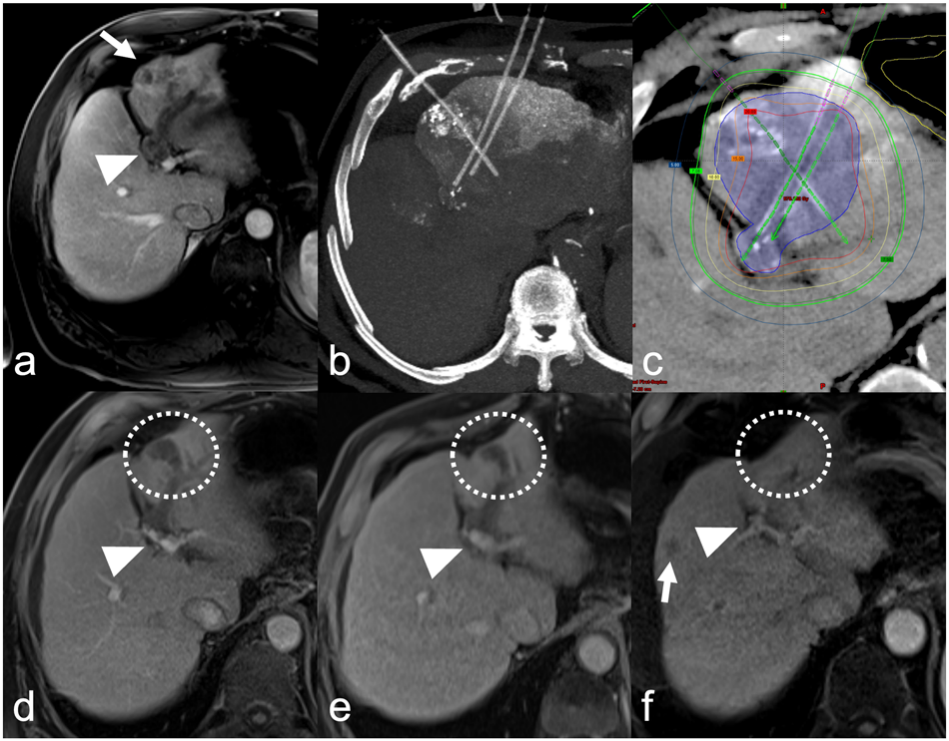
Example images in a 71-year-old male treatment-naive patient with unresectable hepatocellular carcinoma (HCC). a Baseline MRI scan (portal venous phase) shows a single 63-mm HCC (arrow) with portal venous tumor thrombus in the left first-order branch (VP3, arrowhead) in the left liver lobe. b Maximum intensity projection of a computed tomography scan acquired after placement of three brachytherapy applicators and lipiodol deposition after transarterial chemoembolization the day before. c Treatment planning for high-dose-rate irradiation. The region highlighted in blue and bordered by the light blue line represents the 162 mL clinical target volume. The realized minimum dose in the clinical target volume (D100) was 15 Gy. The colored lines around the clinical target volume represent the respective isodose lines: red, 20 Gy; orange, 15 Gy; yellow, 10 Gy; green, 7 Gy; and blue, 5 Gy. Follow-up contrast-enhanced magnetic resonance images at 8 weeks (d), 3 weeks (e), and 12 months (f) show persistent tumor control and progressive tumor shrinkage (dashed circle), while the arrowhead shows portal venous tumor thrombus shrinkage from VP2–3 (d), to VP2 (e) and VP1 (f)
